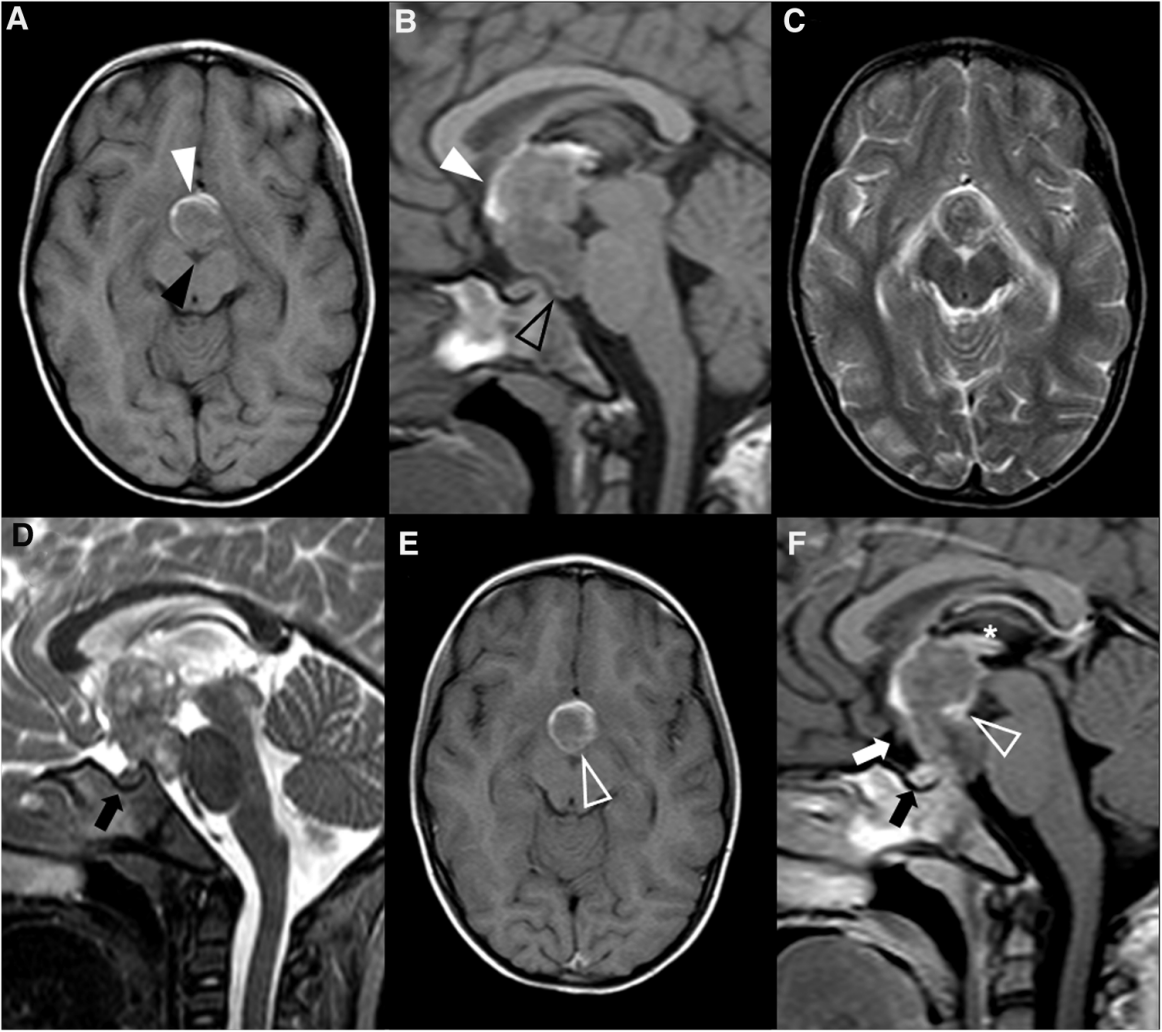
Teratoma in a 4-year-old girl. Brain MR imaging shows an expansile lesion separated from the pituitary gland, centered in the suprasellar cistern with extension into the interpeduncular (black arrowhead) and pre-pontine cisterns (empty black arrowhead). The lesion is mainly isointense on axial (A) and sagittal (B) T1WI, but presents a linear component spontaneously hyperintense anteriorly, that corresponds to adipose tissue (white arrowheads). On axial (C) and sagittal (D) T2WI the lesion shows heterogeneous signal. Post-contrast axial (E) and sagittal (F) T1WI shows only minor posterior peripheral enhancement (empty white arrowheads). There is moderate mass effect over the anterior recesses of the III ventricle (asterisk) and the optic chiasm (white arrow). Note normal positioning and enhancement of the adenohypophysis and absent hyperintense T1 bright spot of the neurohypophysis (black arrows).
